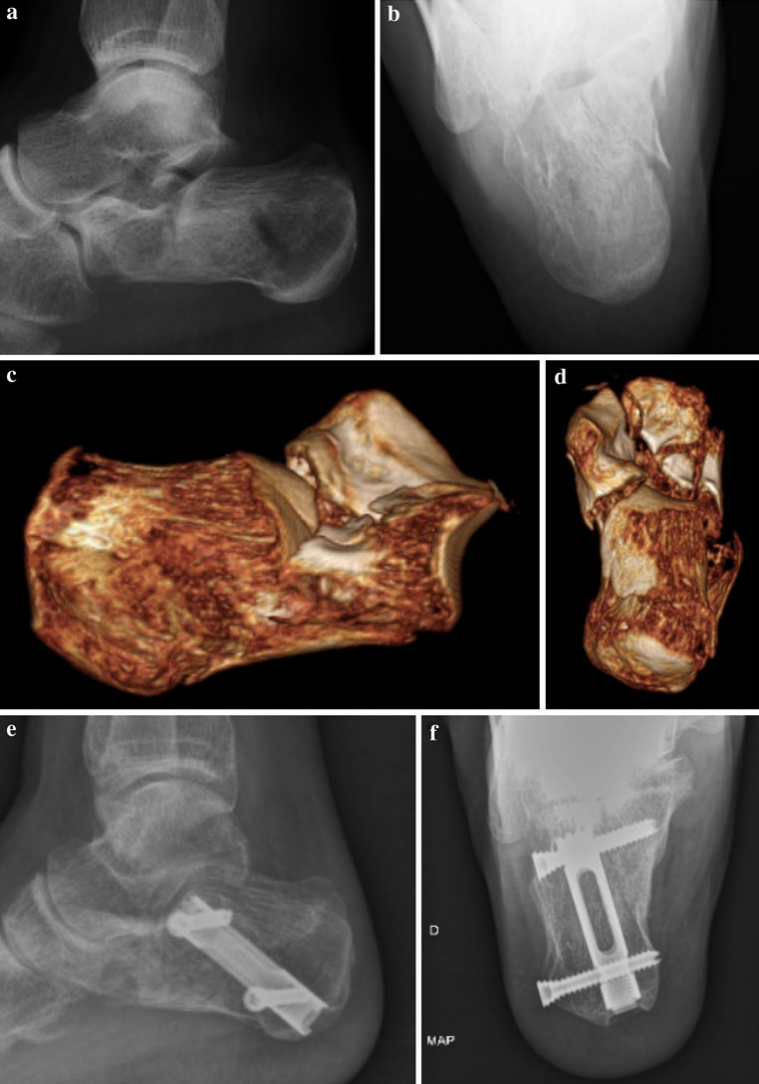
Preoperative and postoperative views of a calcaneal fracture in a 21-year-old woman. a Preoperative lateral view, b preoperative retrotibial view, c, d tridimensional preoperative CT, e postoperative lateral view, f postoperative retrotibial view
